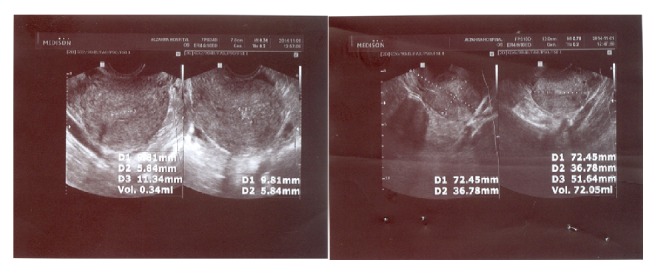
Abdominal ultrasonography view of patient before any treatment protocol.
